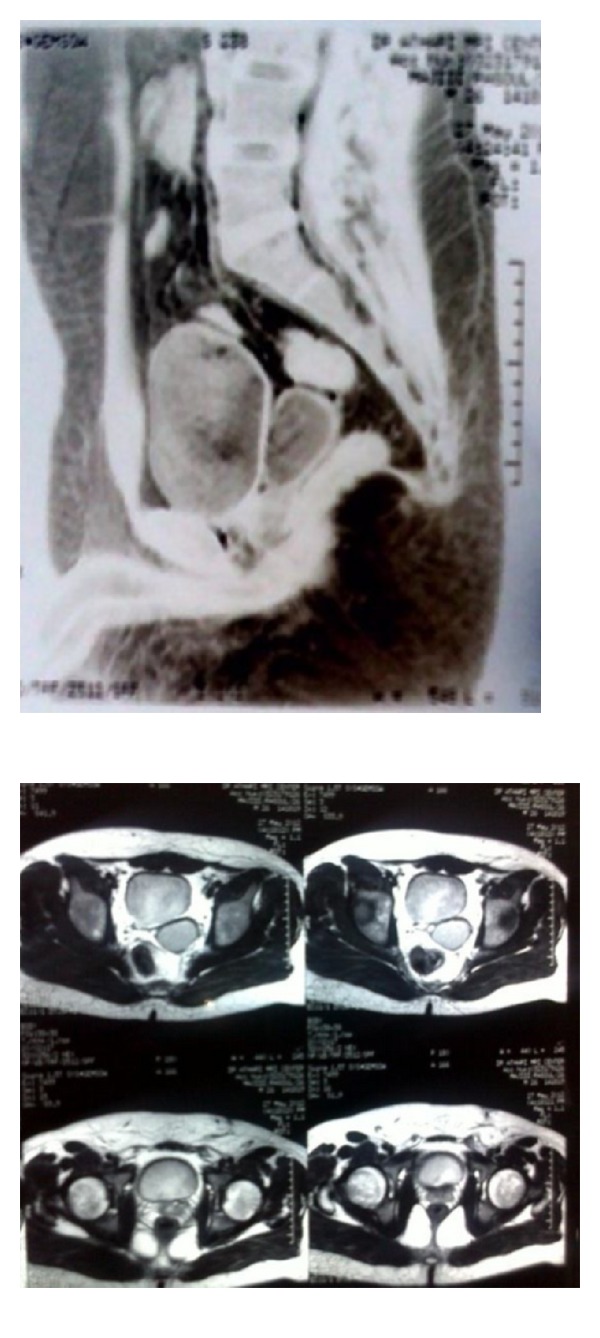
Pelvic MRI showing a large cystic structure in the left seminal vesicle and right seminal vesicle hypoplasia.
